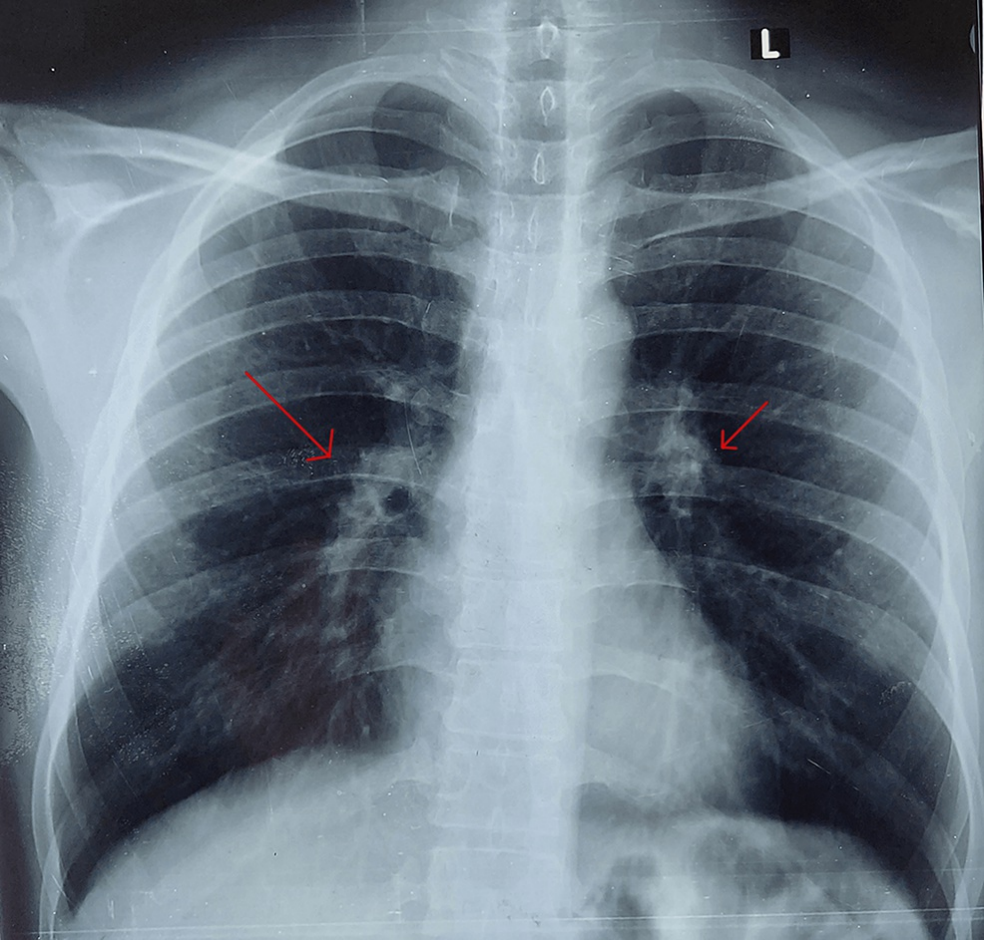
Infiltrations seen on chest X-ray, especially in the right lung and bilateral hilar involvement.
